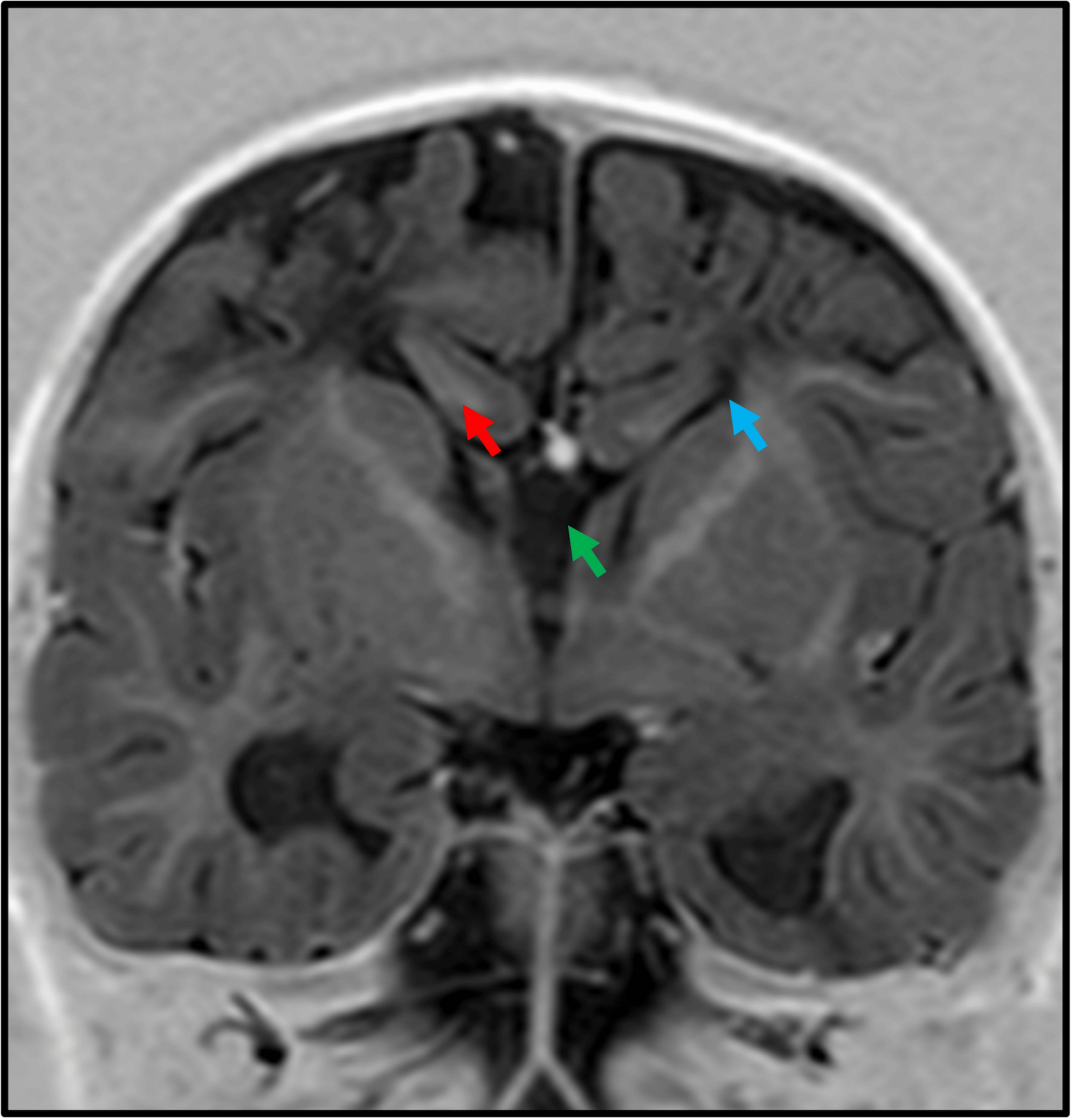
Corpus callosum agenesis with Probst bundle. Coronal T1-weighted inversion recovery image shows bundles of Probst (red arrow) with high riding third ventricle (green arrow). Viking helmet appearance (blue arrow).
